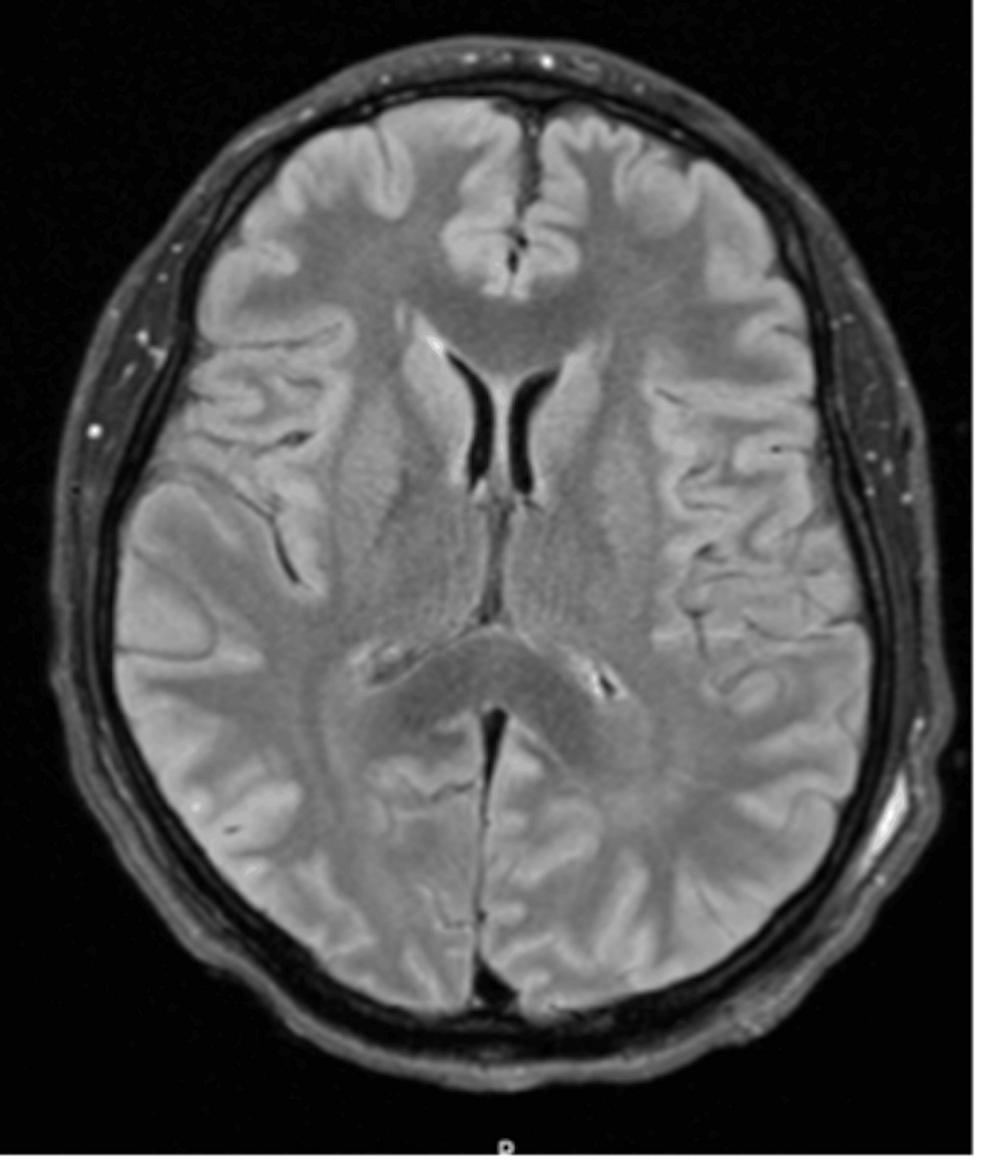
MRI brain T2-FLAIR sequence one day after admission was unremarkable.
